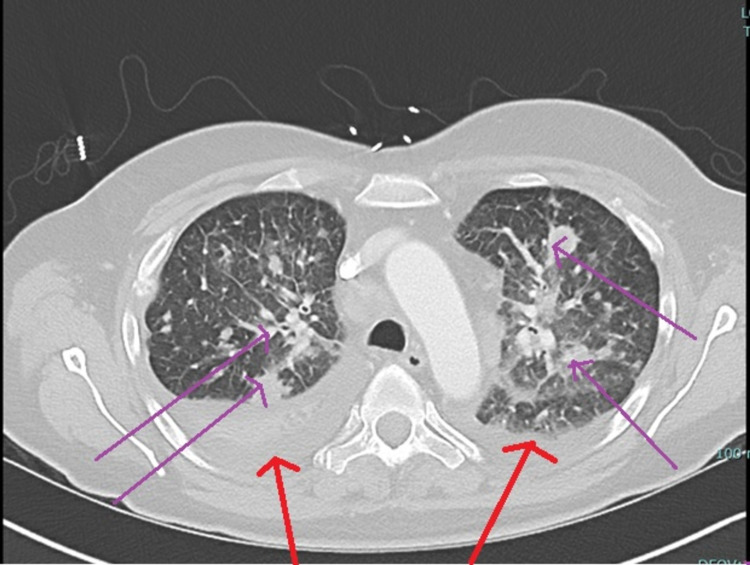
CT of chest, abdomen, and pelvis The purple arrow shows innumerable pulmonary masses and lymphangitic carcinomatosis in bilateral lungs with focal areas of hypoattenuation consistent with necrosis and mediastinal and bilateral hilar lymphadenopathy with mass effect on multiple bronchi. This is consistent with the patient's biopsy-proven metastatic adenocarcinoma. The red arrow shows bilateral pleural effusions and compressive atelectasis of the right lower lobe.
